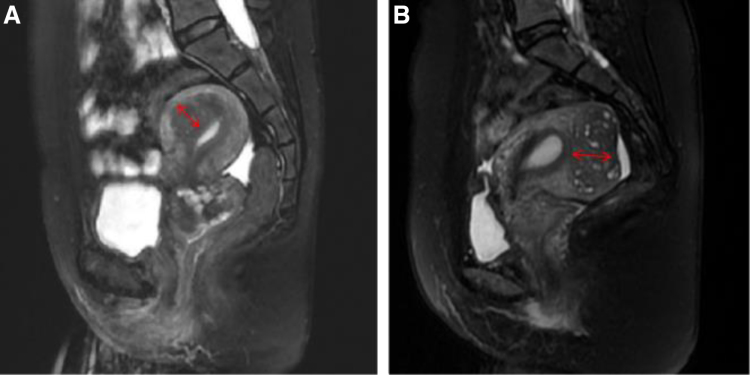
T2 - weighted MRI images of the internal and external ADM. (A) Internal ADM: The maximum junction zone thickness is ≥ 12 mm, and the ratio of the maximum junction to the ipsilateral myometrial thickness is > 40% (red arrow). The outer myometrium is normal. (B) External ADM: The lesion is located in the outer myometrium (red arrow), and there is healthy muscle tissue between the lesion and the junction zone. ADM = adenomyosis, MRI = magnetic resonance imaging.
